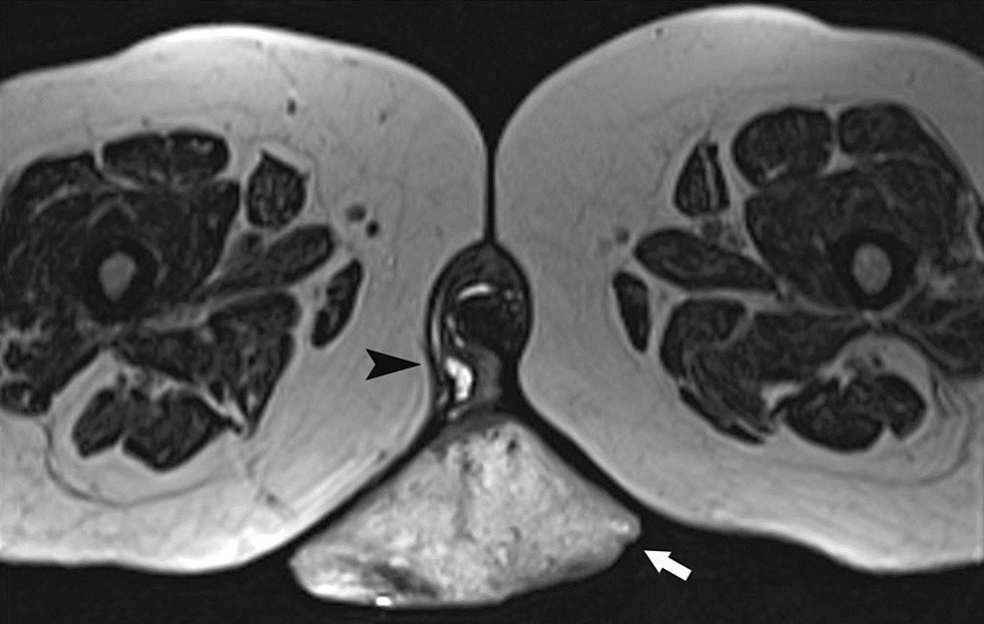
An axial T2-weighted magnetic resonance image shows a large pedunculated mass (arrow) with a stalk (arrowhead) arising from the labia majora on the right
